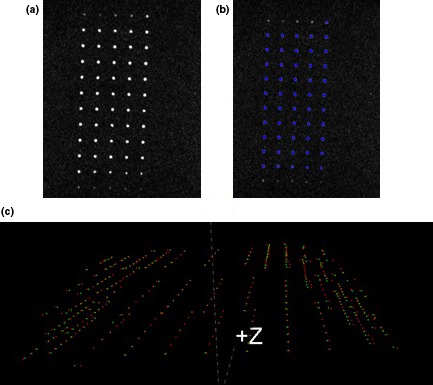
The process for the calculation of the geometric distortion. (a) MR image, (b) Automatic seed generation based on a threshold, and (c) 3D comparison of the MR (red) and CT (green) control points.
